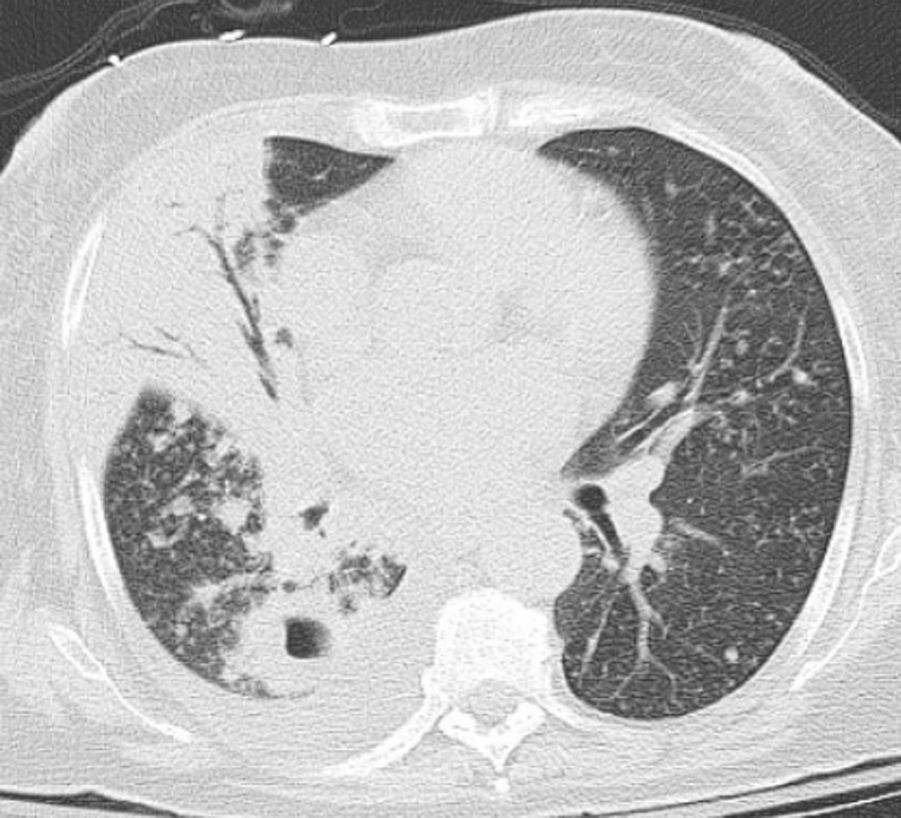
CT chest without contrast demonstrated a moderate-sized right lung infiltrate with multiple bilateral pulmonary nodules and pulmonary masses, some of which were cavitary.
